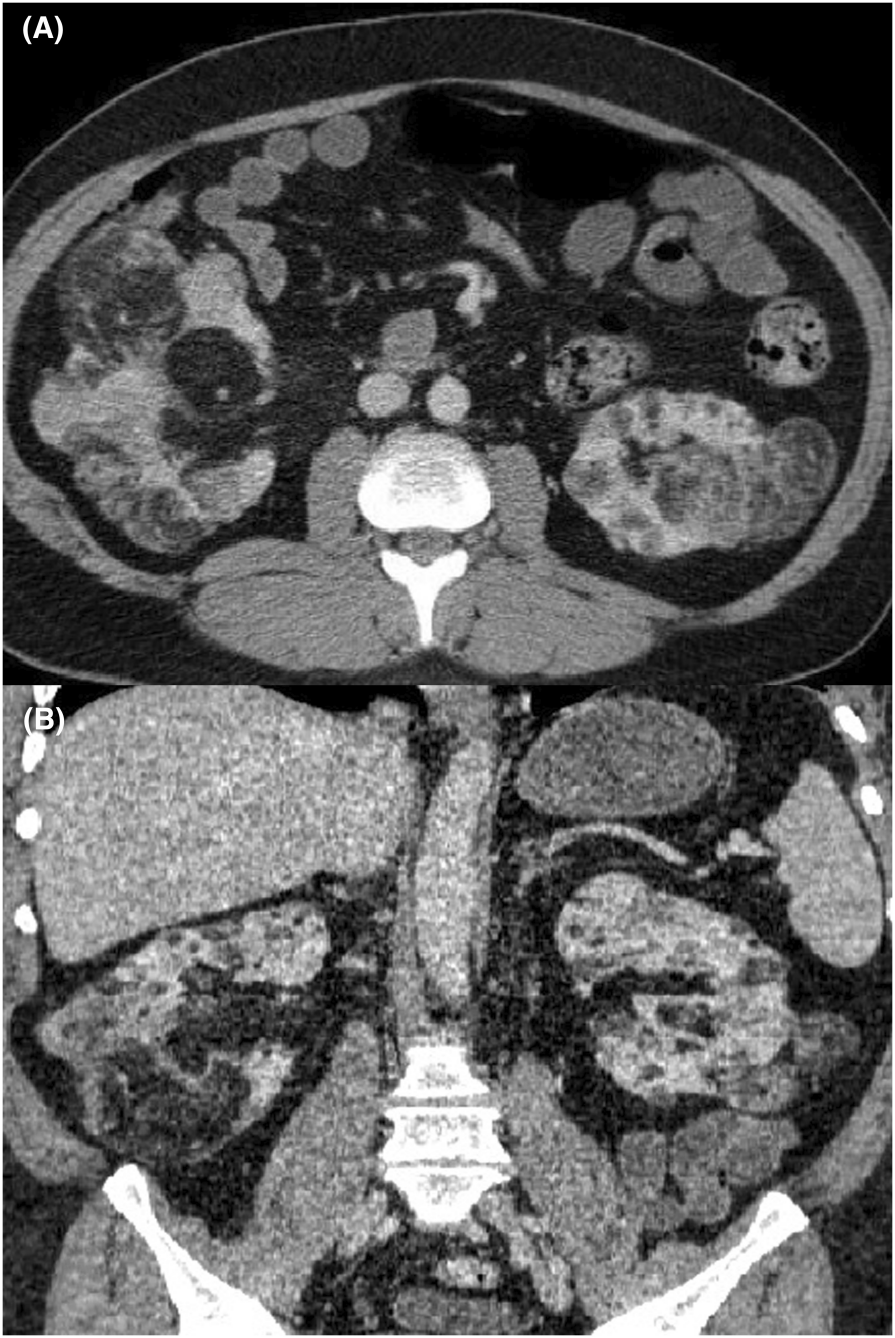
Contrast‐enhanced abdominal CT scan shows multiple renal masses in both kidneys, primarily consisting of adipose tissue in axial (A) and the coronal plane (B), without extension into the renal veins or IVC and with no enlarged lymph nodes.
